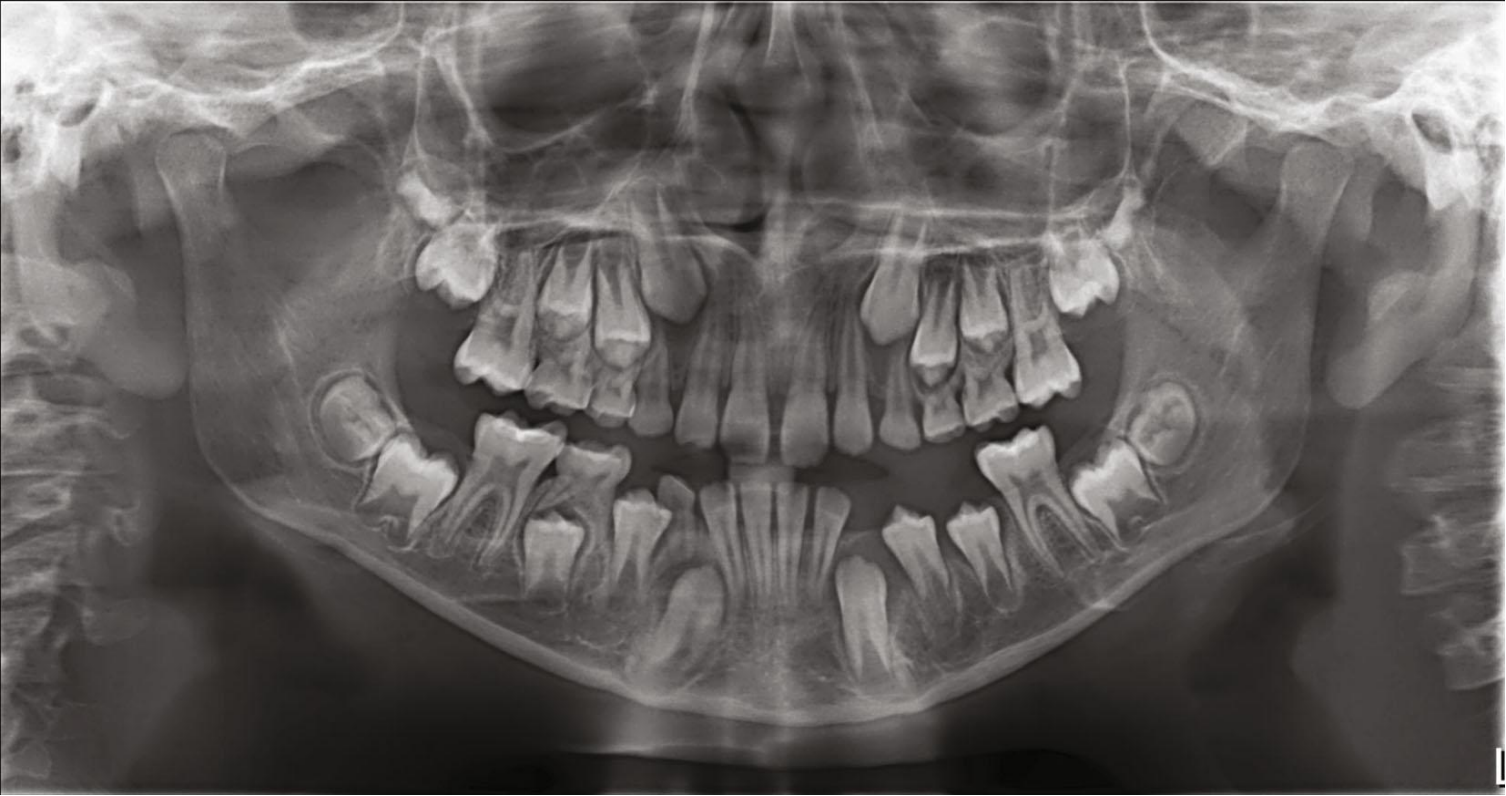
One-year panoramic radiographic follow-up showed the remarkable eruptive progression of the permanent teeth.
